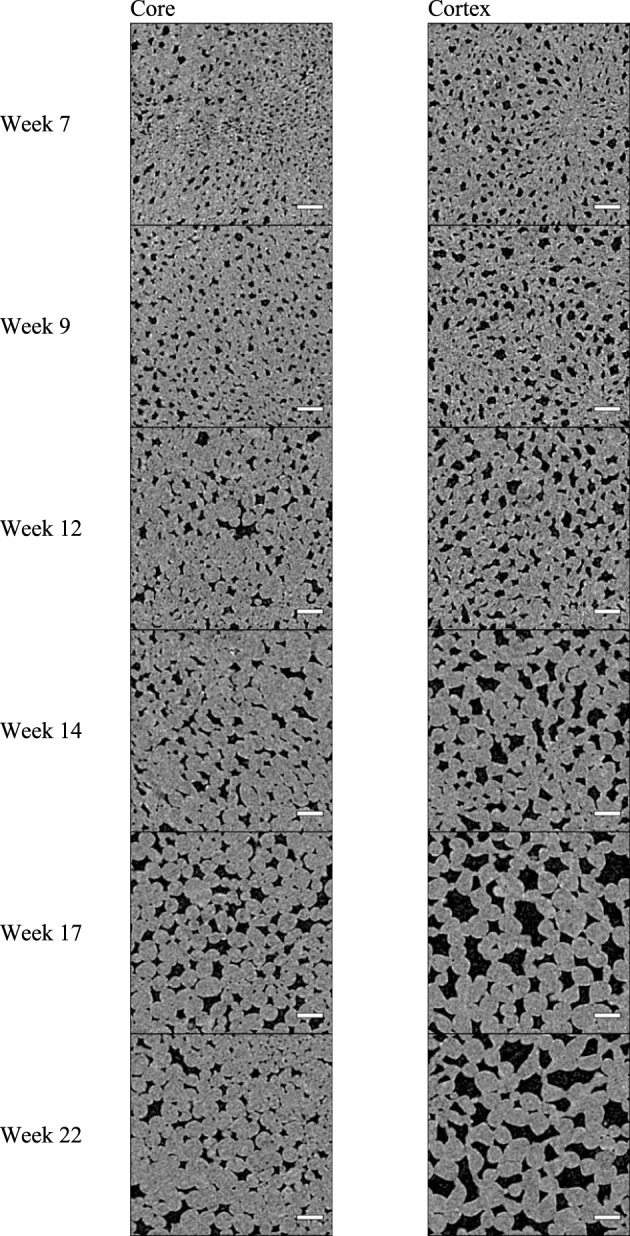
X-ray CT virtual cross-sections through “Jonagold” core and cortex tissue at different times during apple fruit development: 7, 9, 12, 14, 17, and 22 weeks after full bloom), obtained by means of the SkyScan 1172 system. Scale bars indicate 250 μm. Images measure 1.9 × 1.9 mm.
